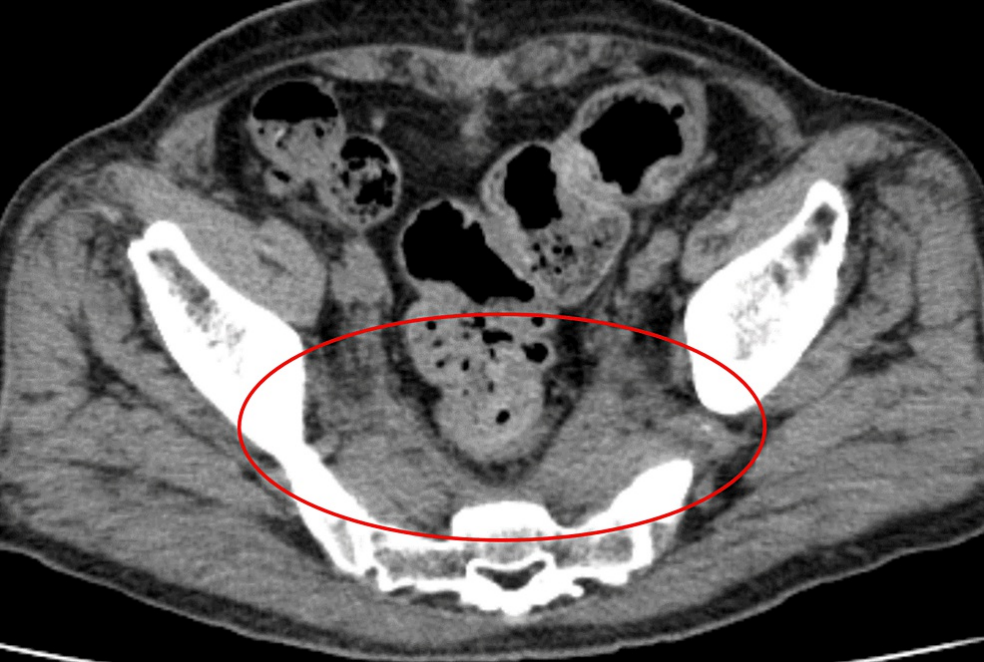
Abdominal computed tomography approximately 1 month after the start of treatment Abdominal computed tomography showed shrinkage of the soft-tissue masses around the ventral sacrum.
